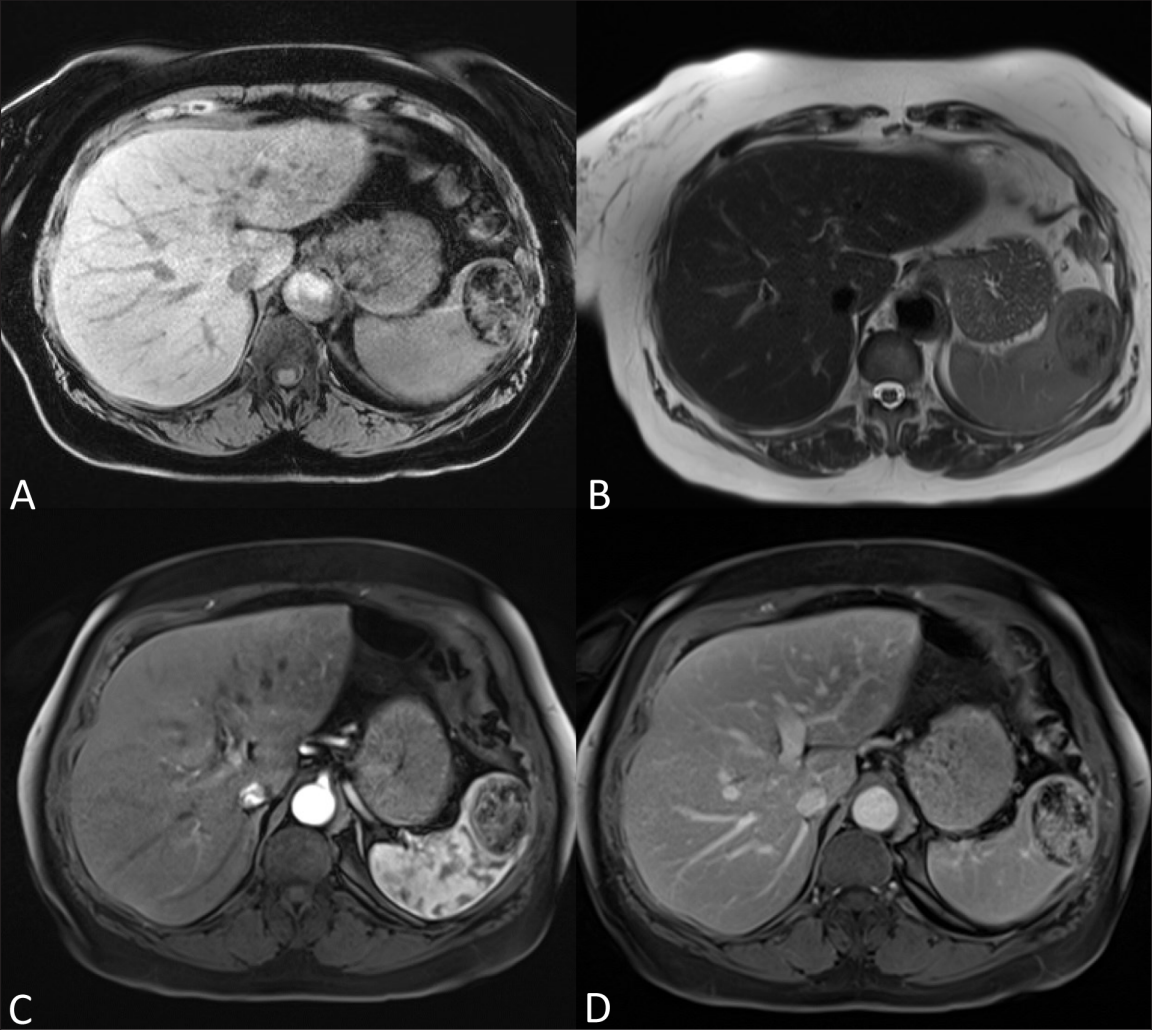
(A) Unenhanced axial fat suppressed T1-weighted imaging shows the lesion is sharply delineated with abundant hypointense foci. (B) Axial T2-weighted imaging shows a sharply delineated heterogeneous mass with both iso- and hypointense components. (C–D) Axial fat suppressed T1-weighted imaging after injection of gadolinium contrast shows a relative heterogeneous and weak enhancement in the arterial phase progressively filling in at the delayed phase, although remaining heterogeneous. No central scar or spoke-wheel enhancement pattern was present.
